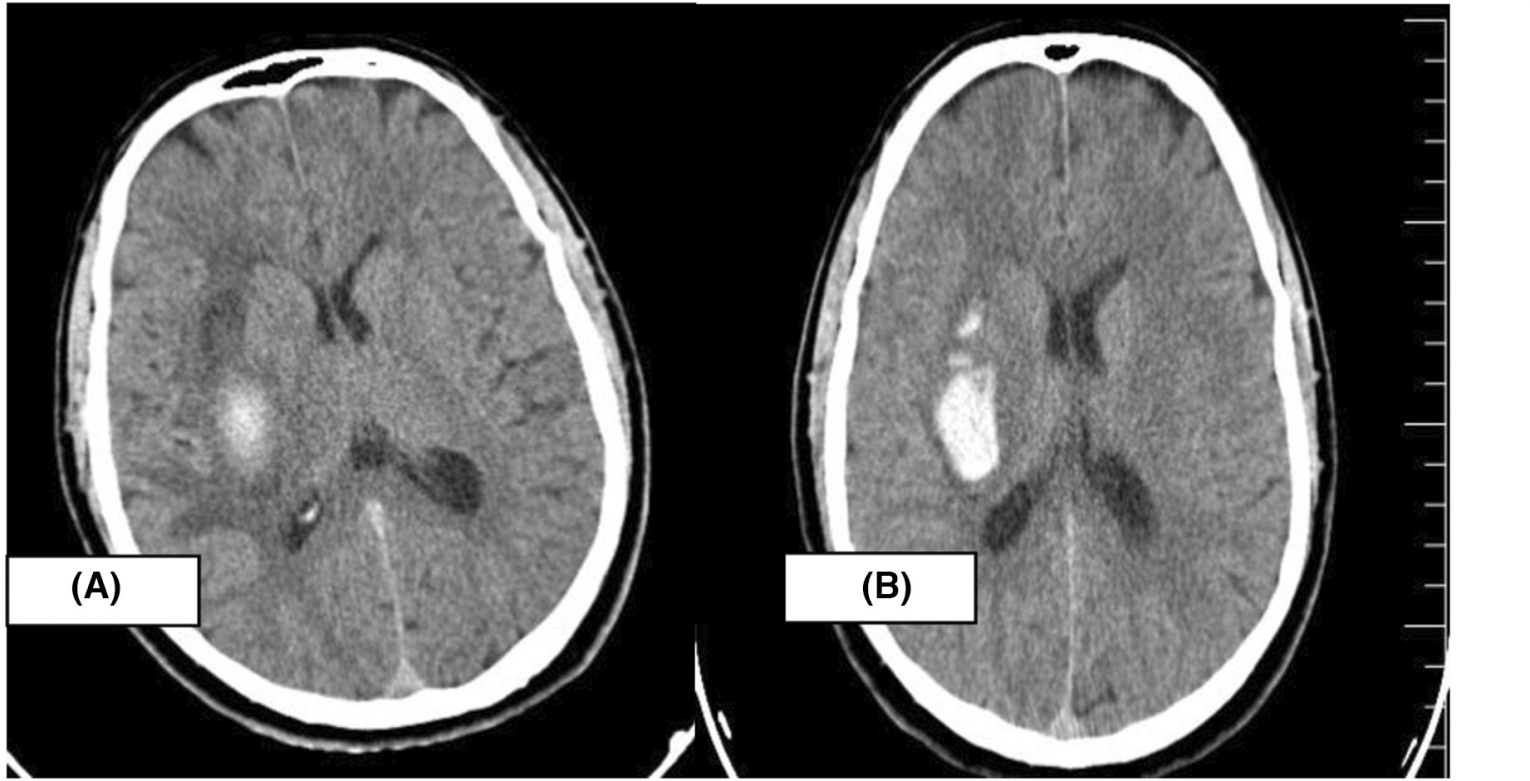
CT head showing slight hematoma resorption of 51 × 22 mm in the right basal ganglia after 5 days.
